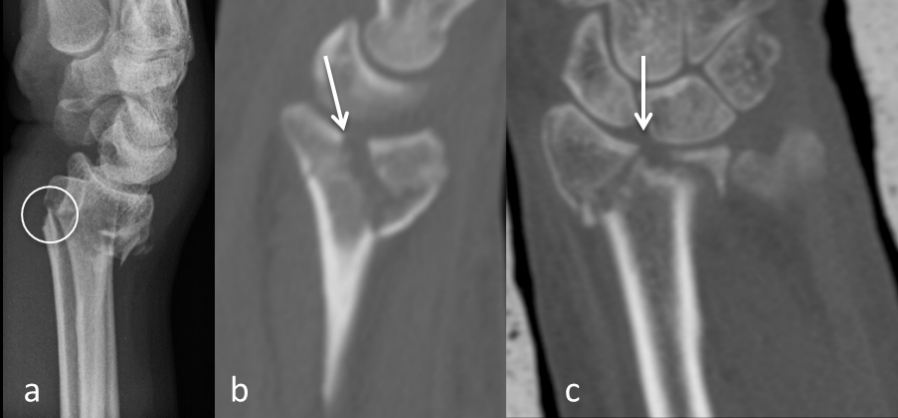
a: The parameter displaced palmar cortex in a conventional sagittal radiograph (circle). b: The parameter displaced frontal split in a sagittal plane of a CT-scan (arrow). c: The parameter displaced sagittal split in a frontal plane of a CT-scan (arrow). The main parameters of the heuristic.
